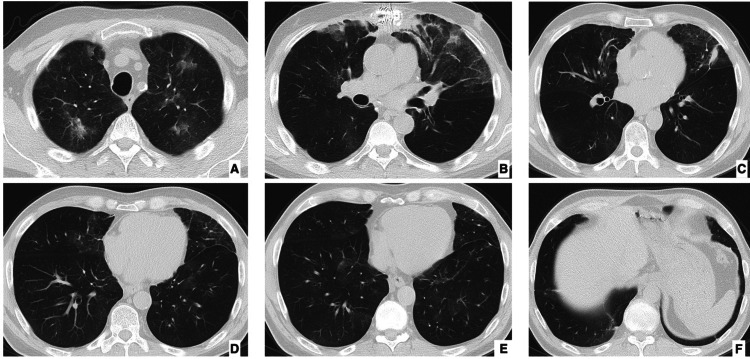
Admission chest computer tomography Axial chest CT images at multiple levels (A–F) showing patchy ground-glass opacities and consolidations with a peripheral and posterior predominance, associated with interlobular septal thickening and vascular enlargement.
